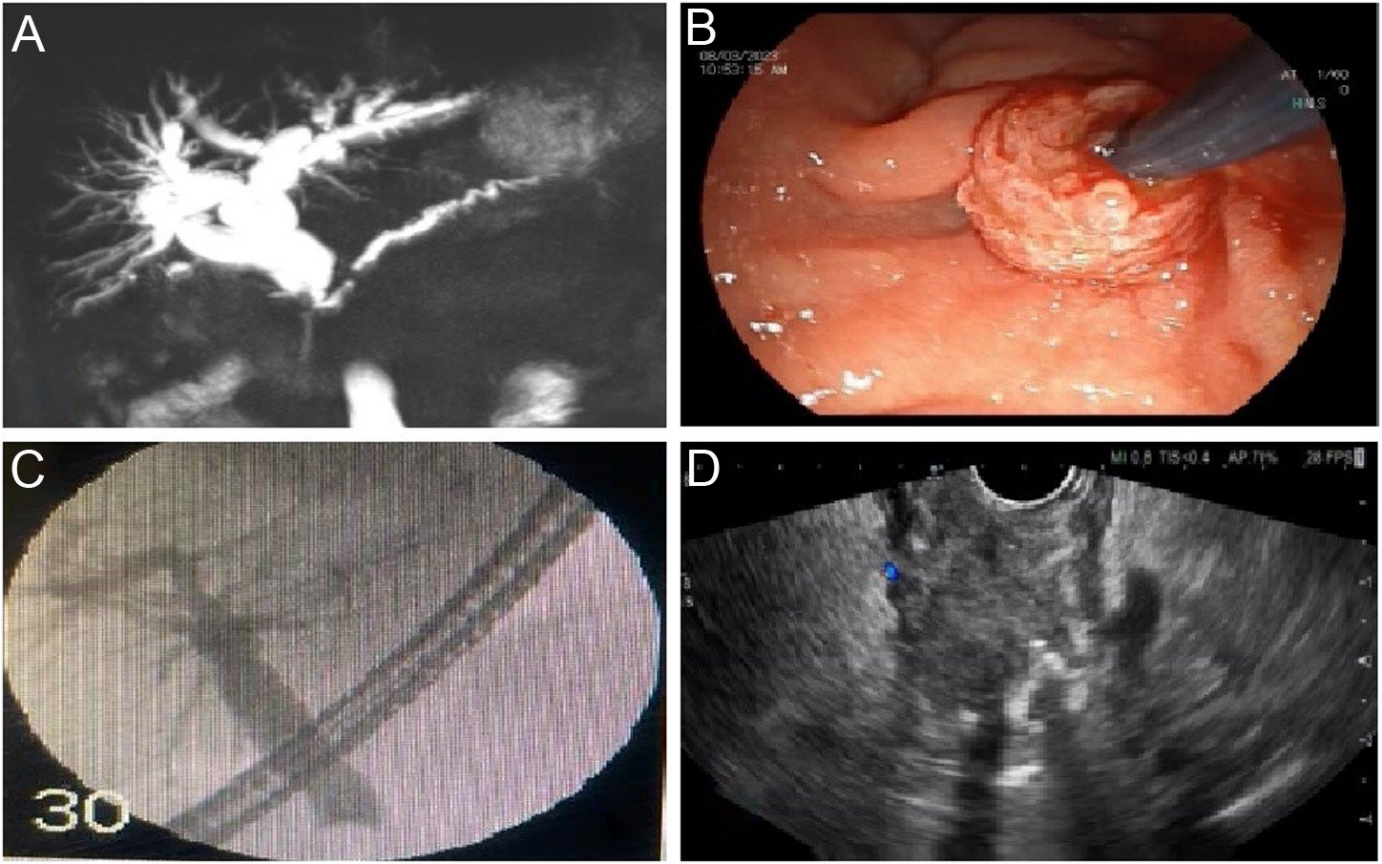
(A) Dilatation in the proximal common bile duct and intrahepatic bile ducts, with a blunt ending in the distal common bile duct as shown in magnetic resonance cholangiopancreatography imaging. (B) Irregular infiltrative involvement around the papilla in endoscopic retrograde cholangiopancreatography. (C) Cholangiography demonstrated dilatation in the common bile duct and intrahepatic bile ducts and blunt ending of the distal common bile duct. (D) Heterogenous hypoechoic lesion with irregular borders in endoscopıc ultrasonography.
